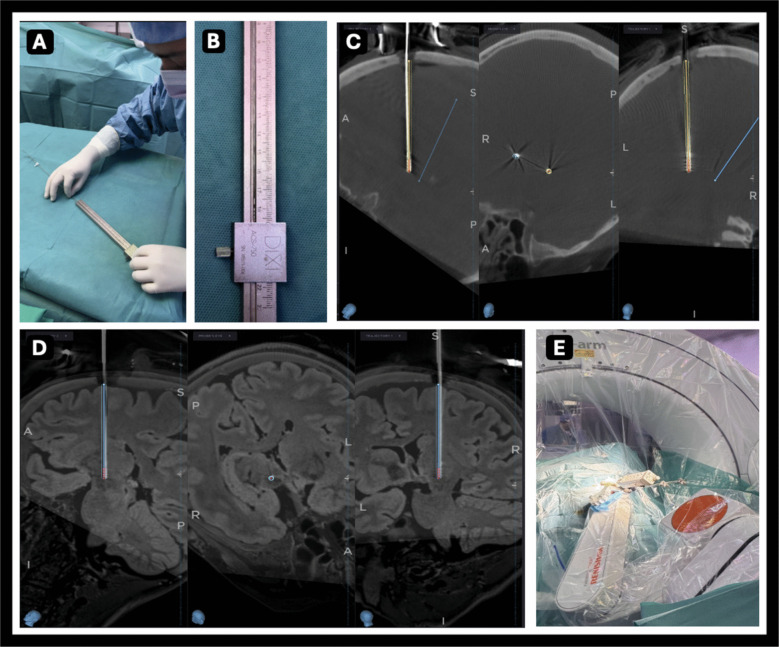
Robot-guided trajectory execution and depth confirmation. A Guide cannula preparation before insertion. B Identification of the manufacturer’s orientation marker on the directional DBS lead to ensure correct rotational alignment before implantation. C Intraoperative CT confirming stereotactic alignment of the trajectory. D MRI fusion demonstrating final anatomical target verification. E Sterile positioning of the robotic arm and O-arm system during trajectory execution and imaging acquisition
